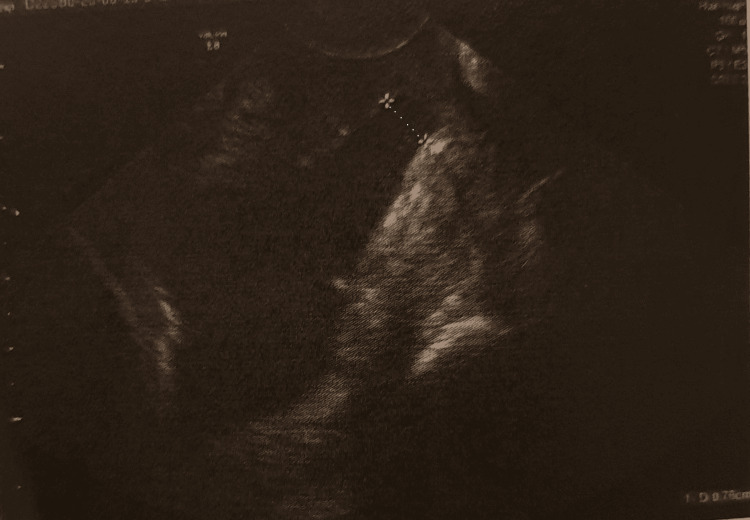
Ultrasound image The image shows cervical dilation at 7.6 mm.
